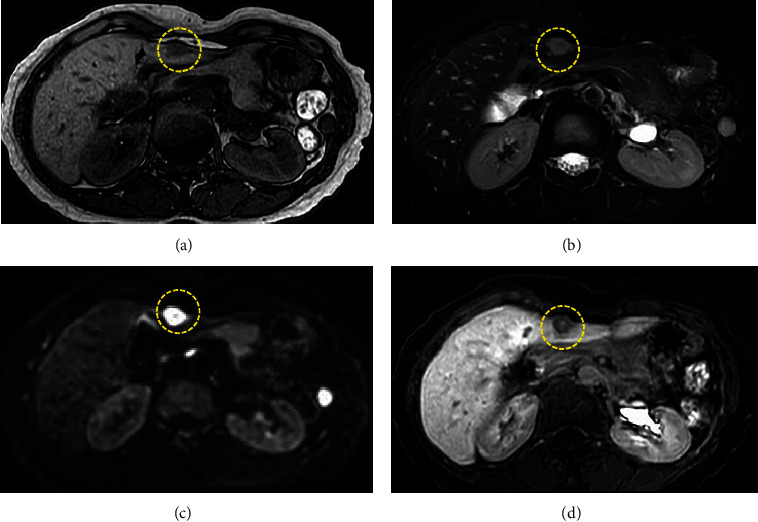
MRI findings. (a) The mass was visualized as low signal in T1WI. (b) The mass was visualized as high signal in T2WI. (c) On diffusion-weighted image, the mass was shown as high signal intensity. (d) On gadolinium ethoxybenzyl diethylenetriamine pentaacetic acid-enhanced MRI, the mass was shown as low signal intensity in hepatobiliary phase (yellow-dotted area).
